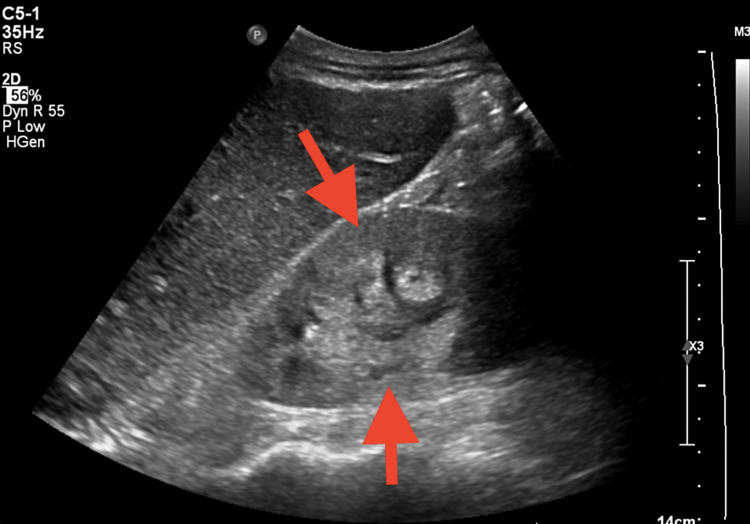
Renal ultrasound showing kidneys with size and parenchymal thickness within normal limits but increased echogenicity, suggesting medical renal disease (arrows) The right and left kidneys measure 10.6 x 5.2 x 4.5 cm and 10.6 x 4.6 x 3.8 cm, respectively. Both kidneys are increased in echogenicity suggesting medical renal disease. No hydronephrosis, shadowing renal calculus, or perinephric fluid collection.
